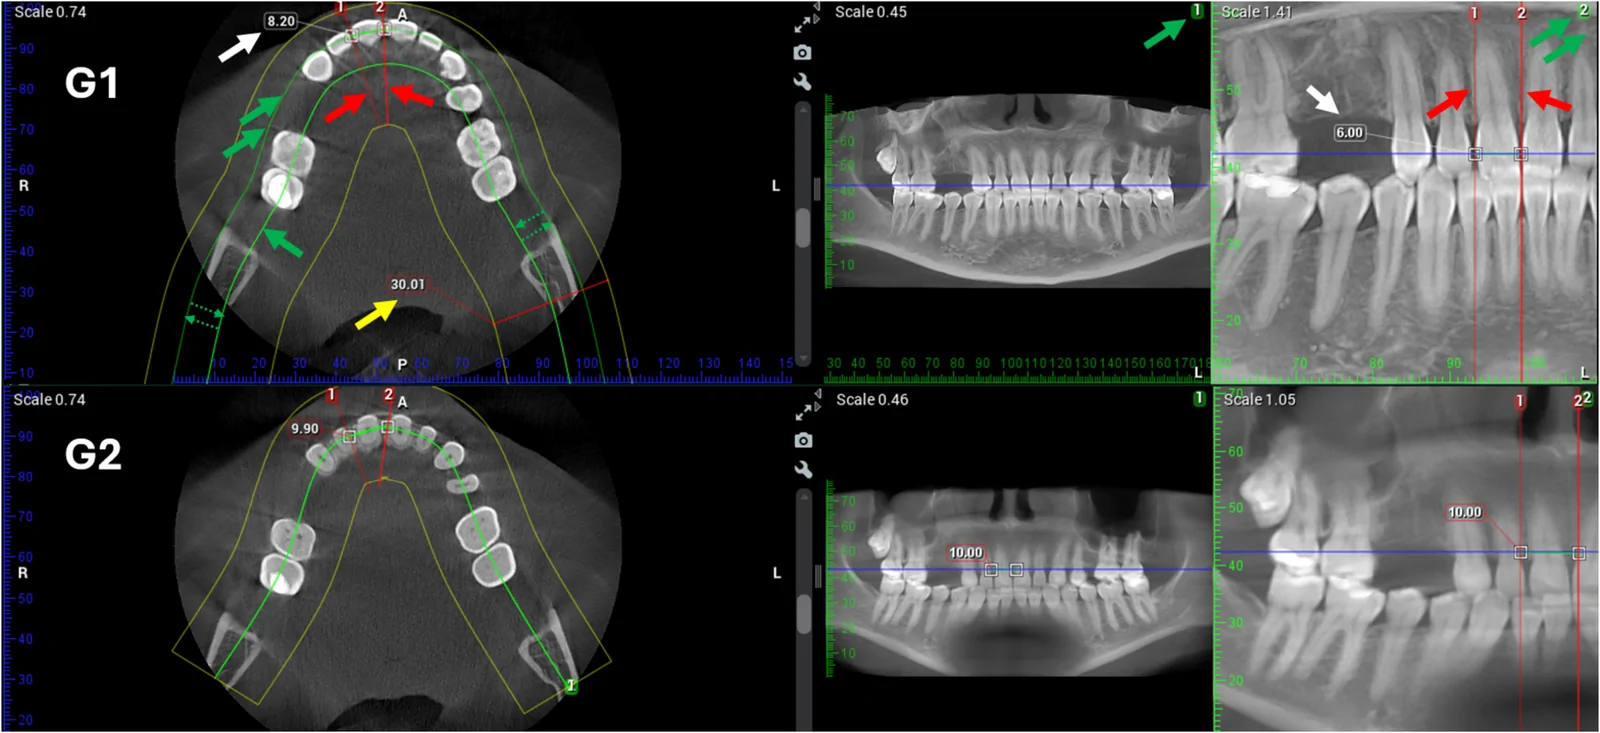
G1: Crown-level measurements on the axial view shown on the left (white arrow) and the corresponding reformatted panorama shown on the right (white arrow). Measurement endpoints were adjusted using the fine-tuning handles (end boxes). The red measurement line, measuring 30.01 mm, pointed to by the yellow arrow, indicates the automatically done panoramic contour thickness, which is the distance between the outer and inner curved yellow lines marking the lingual and labial limits of the reconstruction thickness. Note the difference in thickness between G1 and G2. One of the two green curved lines indicates the center of the panoramic curve, where panoramic curve points are drawn either manually or automatically (single green arrow in axial), and is referred to as panoramic slice 1 (single green arrow in panorama). The secondary curved green line (two green arrows in axial) passes through the measurement site (labial to the centerline) and is referred to as panoramic slice 2 (two green arrows in panorama). The distance between the two green curved lines is the panoramic interslice distance or PISD (dotted green arrows). The two red arrows refer to the reference lines used to accurately place measurement points at the intersection of two lines. Note that the red reference lines are converging palatally in the axial slice. Measurements are 8.2 mm on the axial and 6 mm on the reformatted panorama for the same anatomic location (more than 2 mm difference). G2: Bone-level measurements shown on the axial CBCT image and the corresponding reformatted panoramic image. The primary panoramic line and the secondary line coincide (shown as one line, unlike the upper row, which is G1), indicating alignment or matching of the measurement plane with the center of the panoramic curve. Note the false bone defect visible in the lower arch at the mental region. Measurements are 9.9 mm on axial and 10 mm on panorama (nearly identical)
